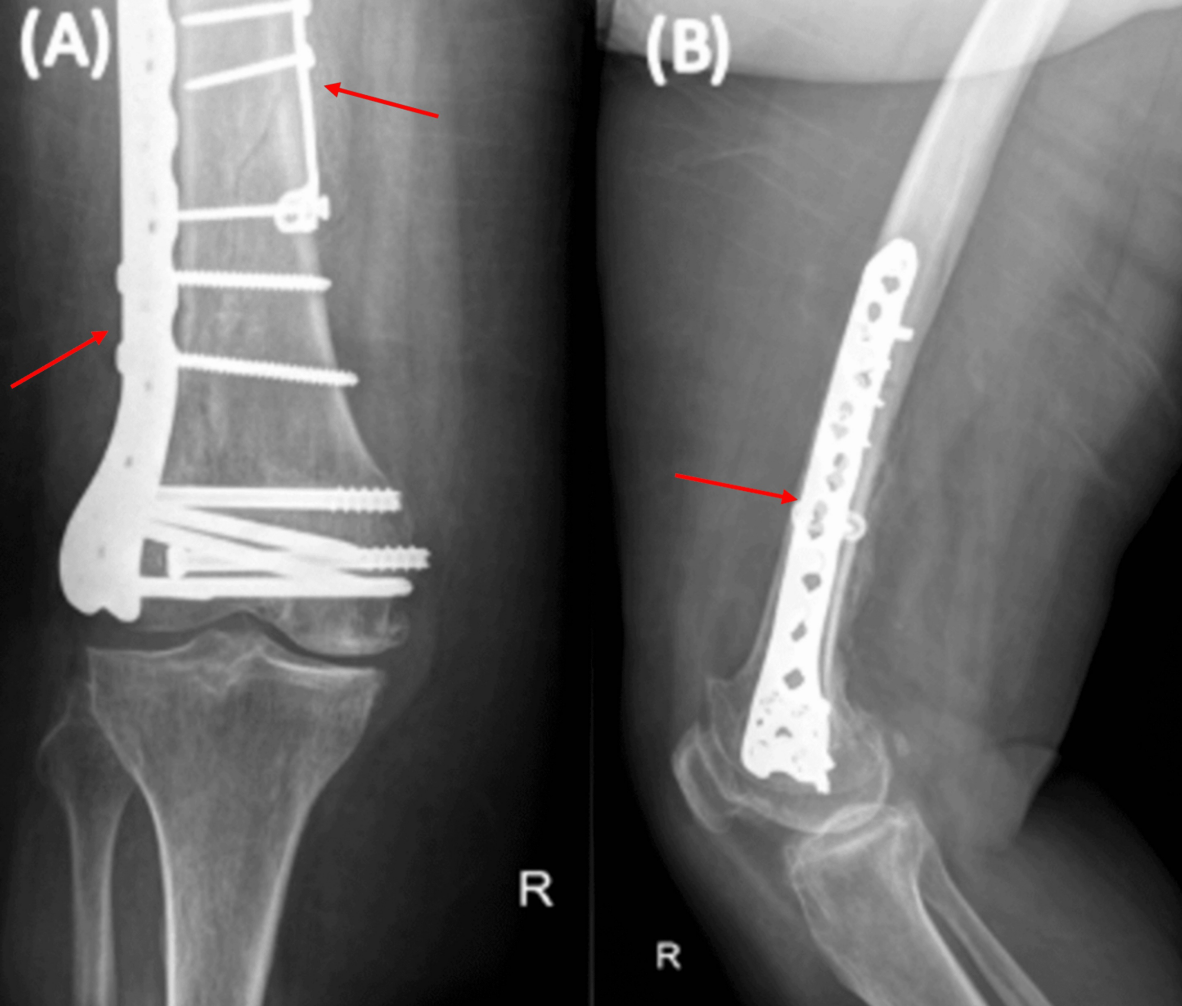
Three-month postoperative X-rays (A) AP and (B) lateral views of the right knee joint. Three-month postoperative photos show screws and plates (red arrows) used for internal fixation to stabilize the distal femur fracture. This technique is used to ensure proper alignment and healing of the bone after a complex fracture.
